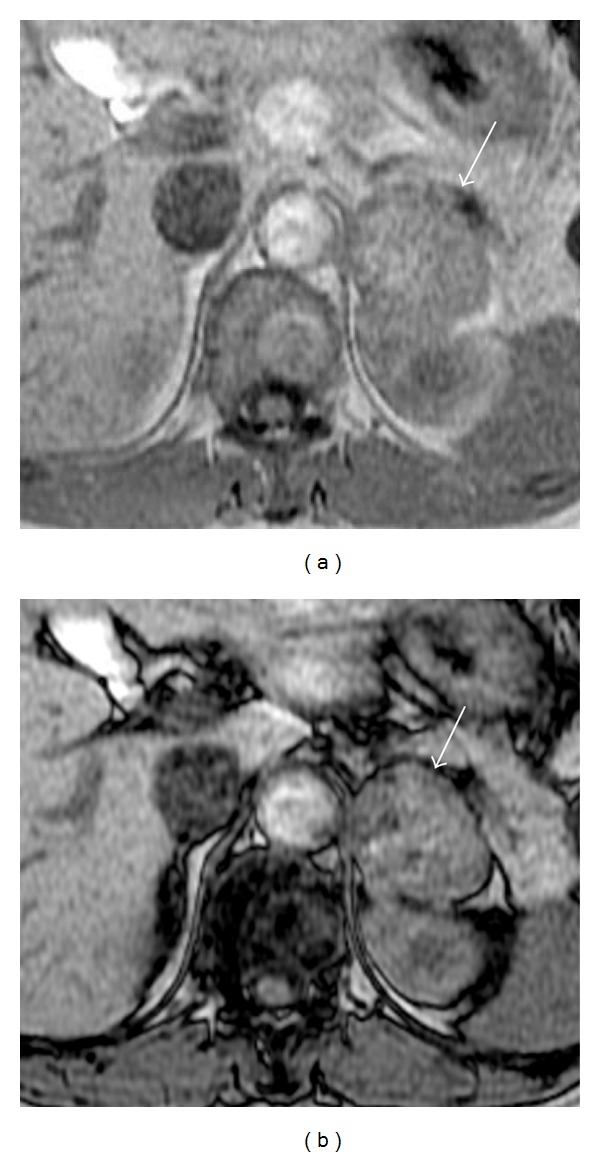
In-phase gradient echo MRI demonstrated a 40 mm, well-defined left adrenal mass (a) (arrow). Opposed-phase imaging showed signal decrease in part of the mass (b), indicating lipid content.
